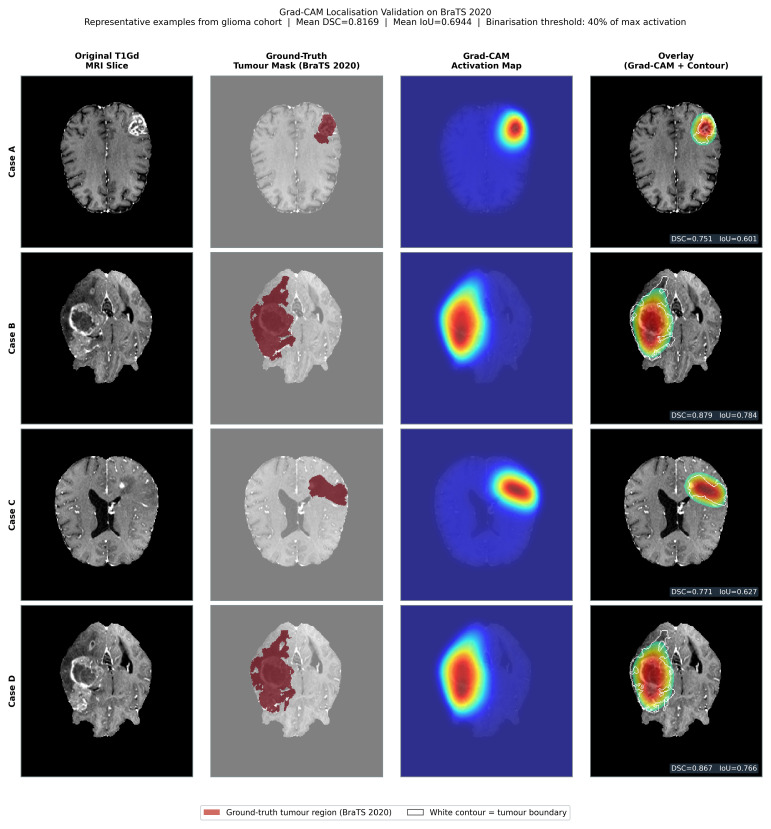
Representative Grad-CAM localisation examples on BraTS 2020 glioma cases showing correspondence between activation regions and tumour masks. Each row corresponds to one representative axial slice from the BraTS 2020 glioma cohort (Cases A–D; all HGG). Column 1: Original T1Gd MRI slice (percentile normalised). Column 2: BraTS 2020 ground-truth tumour region (red overlay). Column 3: Grad-CAM activation map (jet colourmap; binarisation threshold 40% of maximum activation). Column 4: Overlay of Grad-CAM on MRI with white tumour contour; per-slice DSC and IoU annotated. The spatial correspondence confirms that BrainFusionNet consistently attends to diagnostically relevant tumour locations.
